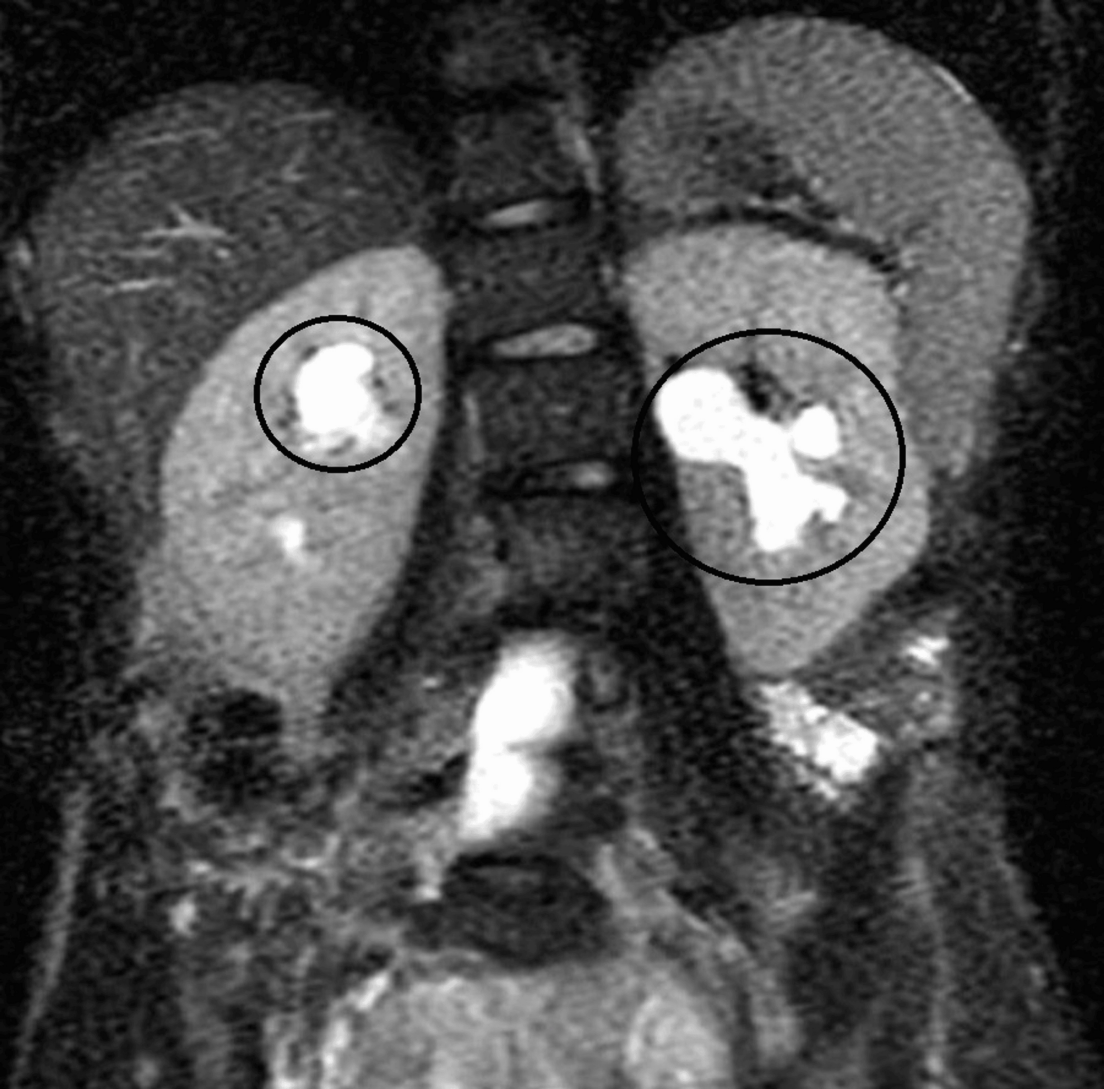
Coronal view of the MRI of the kidneys showing presence of hydronephrosis. Dilated renal calyces are circled.
